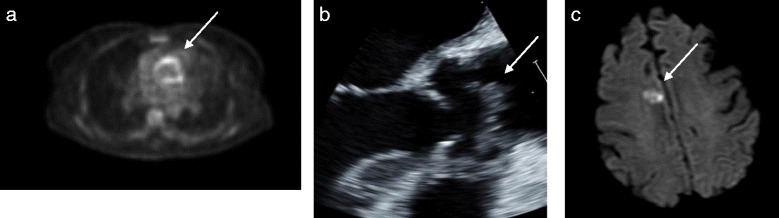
Diagnostic imaging of *Lomentospora prolificans* endocarditis. a FDG-PET axial image showing abnormal linear uptake in the aortic root and the ascending aorta and b Transoesophageal echocardiogram (still shot) axial view demonstrating a large pedunculated mass within the ascending aorta arising from the commissure of the right and non-coronary cusp of the aortic valve with a calcified base; and cerebral emboli c MRI brain axial view, with some motion artefact, showing post-gadolinium enhancement of the right parasagittal frontal lobe consistent with an embolic lesion
